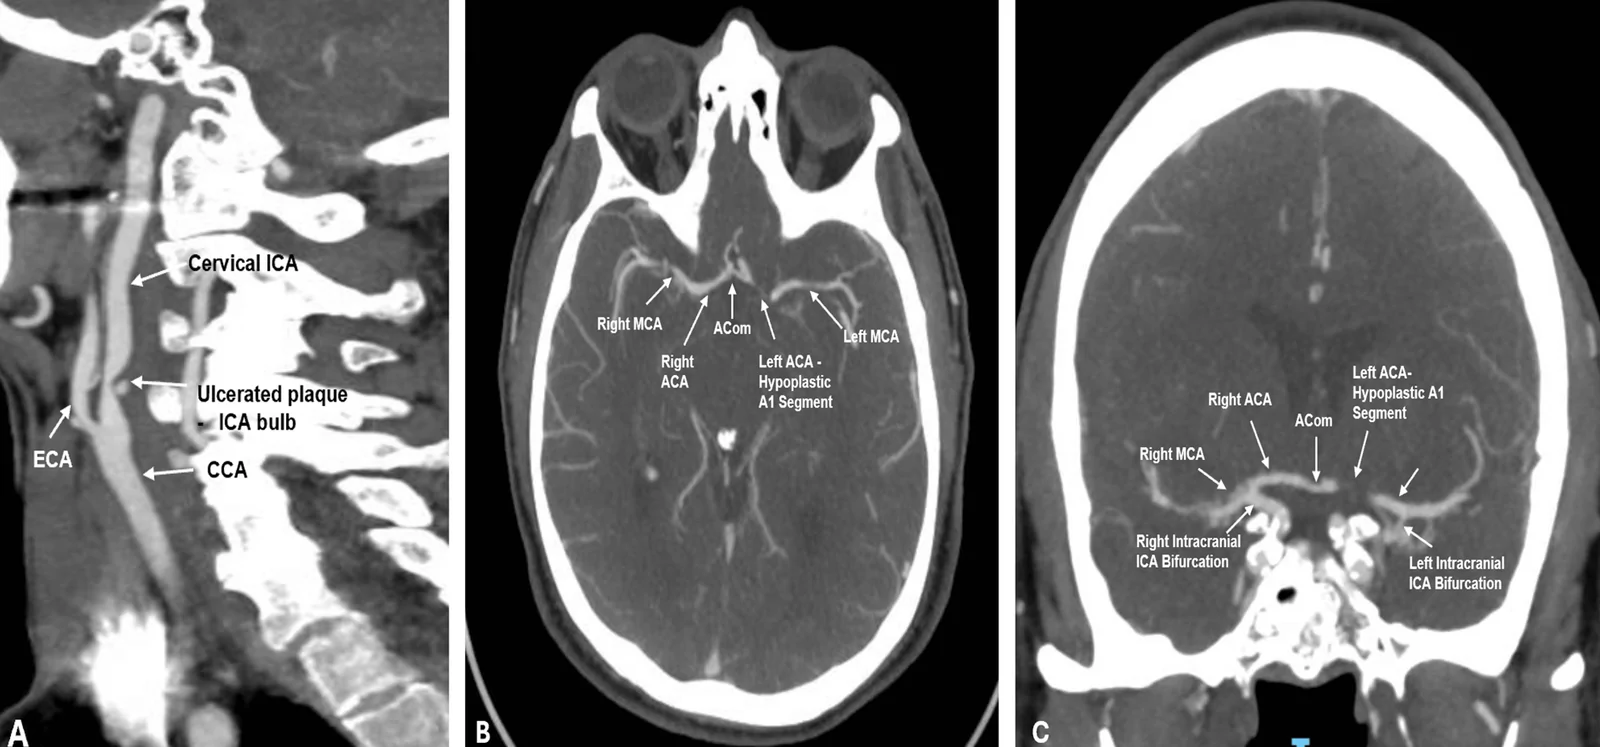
Computed Tomography Angiography Demonstrating Right Cervical Internal Carotid Artery Stenosis With Plaque Ulceration and a Hypoplastic Left A1 Segment. (A) Sagittal CT angiography of the neck demonstrating an ulcerated plaque involving the right proximal cervical internal carotid artery (ICA), resulting in 72% stenosis by North American Symptomatic Carotid Endarterectomy Trial (NASCET) criteria, with a minimal residual luminal diameter of 1.2 mm and a distal reference ICA diameter of 4.3 mm. (B) Axial CT angiography of the head demonstrating marked asymmetry of the A1 segments and a hypoplastic left A1 segment. (C) Coronal CT angiography, on which the A1 segments were best visualized, demonstrating a left A1 diameter of 0.87 mm compared with 2.40 mm on the right. ACA, anterior cerebral artery; ACom, anterior communicating artery; CCA, common carotid artery; ECA, external carotid artery; ICA, internal carotid artery; MCA, middle cerebral artery.
